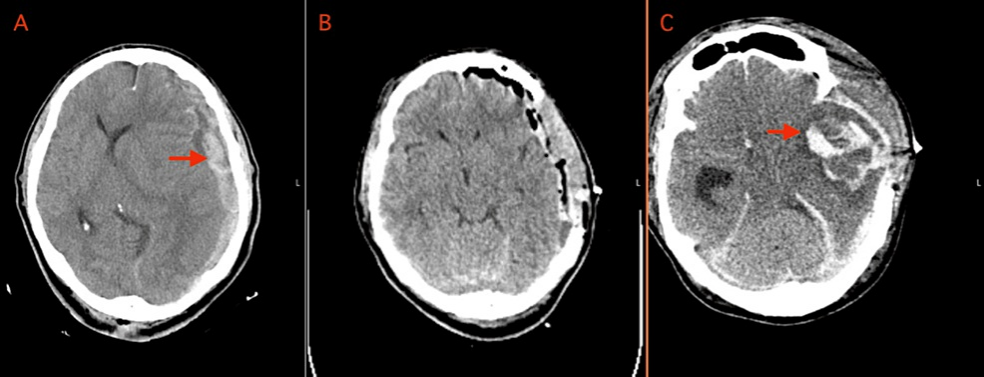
CT images demonstrating DTICH type 1A The left image (panel A) is the initial CT scan on POD 0 for a patient who eventually developed DTICH type 1A. The patient was found to have a massive left-sided acute subdural hematoma (red arrow in panel A) with 2 cm midline shift on the initial trauma evaluation. The middle image (panel B) is a CT scan for the same patient on POD 1 after undergoing left craniectomy. The right image (panel C), a CT scan for the same patient on POD 7, shows a large intracerebral hemorrhage (red arrow in panel C) with effacement of the basal cisterns. CT: computerized tomography; POD: post-operative day; DTICH: delayed traumatic intracerebral hematoma
